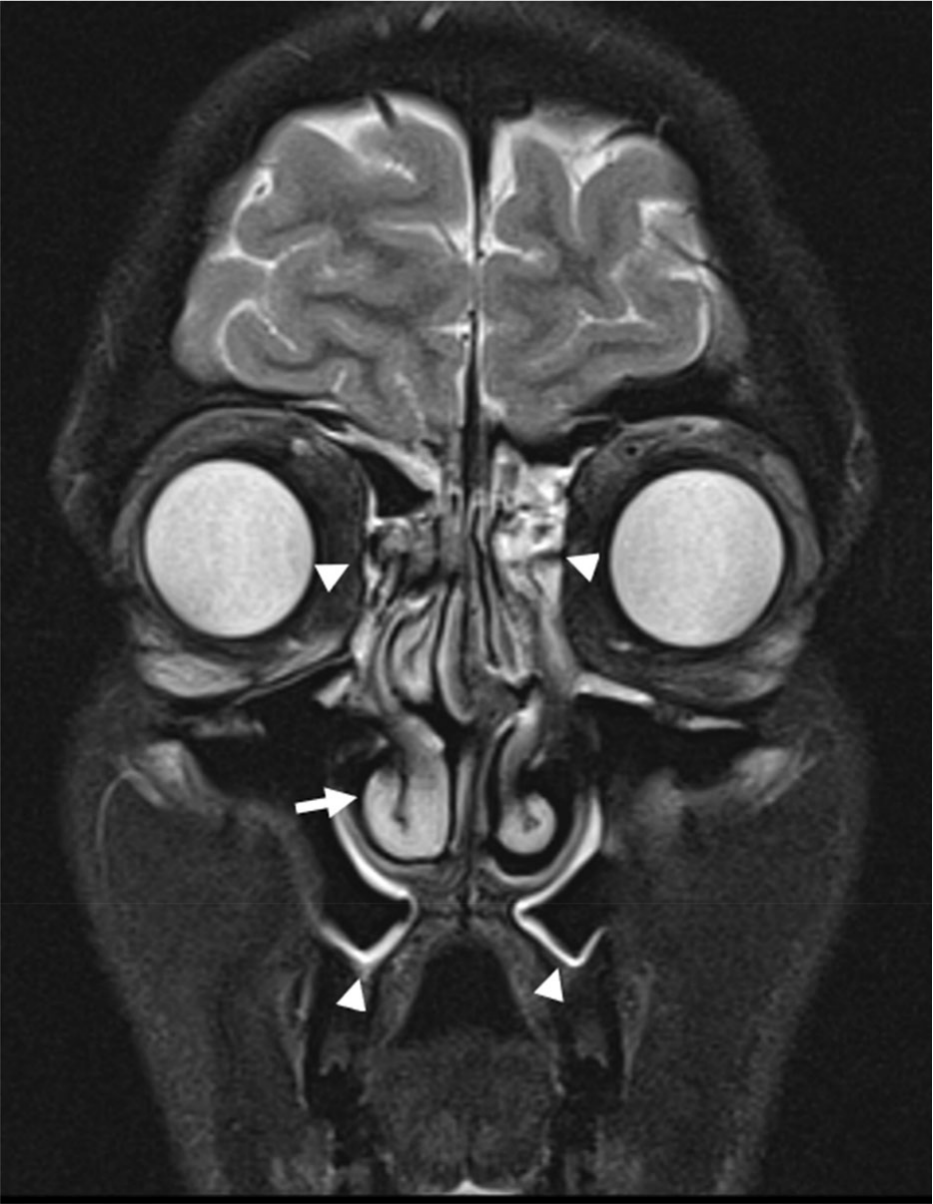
Coronal view of T2 weighted MRI brain and orbits in a 24‐year‐old male patient with GPA showing right inferior nasal turbinate hypertrophy (arrow) and mucosal thickening in the maxillary and ethmoid sinuses (arrowheads).
